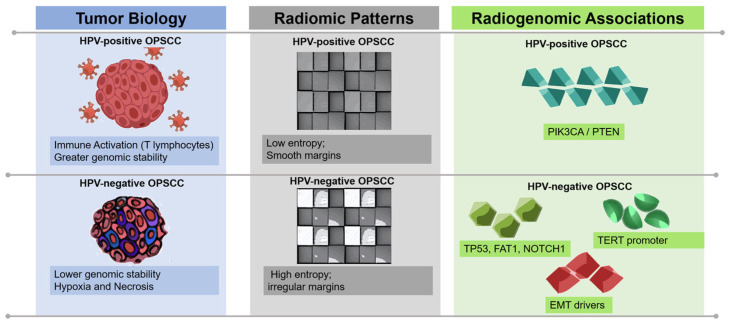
Integrated radiogenomic ecosystem of OPSCC. Biological programs shape multimodal imaging phenotypes across CT, MRI, PET/CT and CBCT. These signatures have been reported to correlate with underlying tumor behavior and support key radiogenomic predictions, including HPV status, aggressiveness and treatment response.
